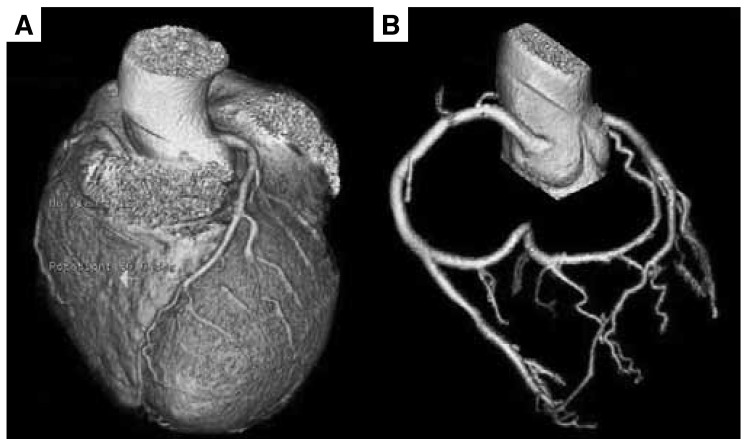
The circumflex artery is absent and only the left anterior descending artery is seen in the multi-detector computed tomography. (A) The left circumflex artery is absent in this view and the left anterior descending artery is clearly visualised. (B) A super-dominant right coronary artery is seen. The terminal portion of the right coronary artery unusually continues in the region of the left circumflex artery.
